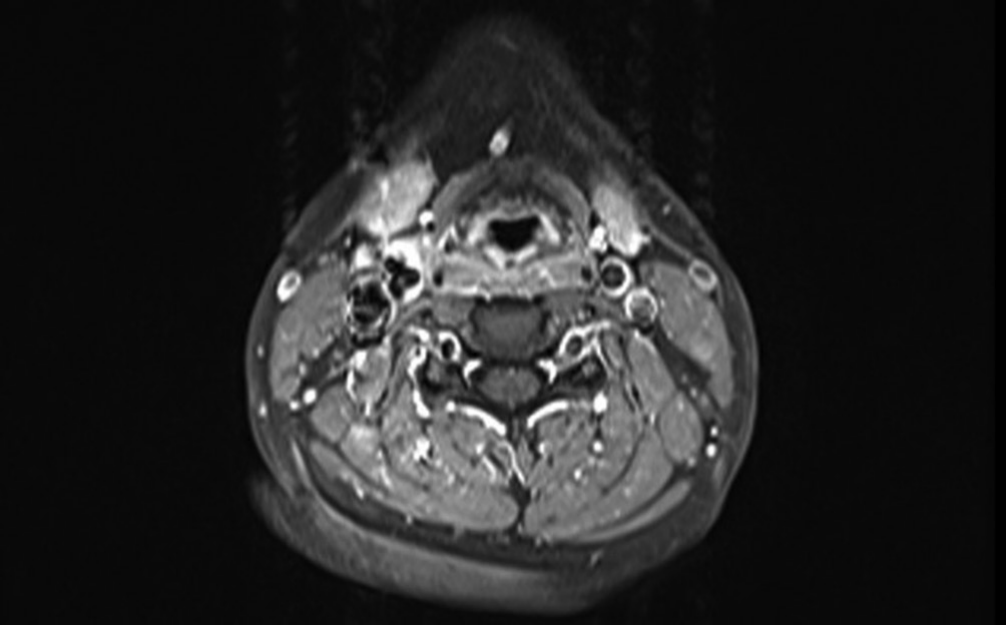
Post contrast *T*_1_ weighted MR image of the neck showing pericarotid enhancement at the level of bifurcation of carotid artery.
